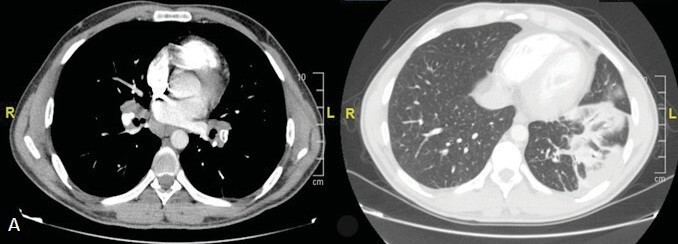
Pulmonary CT angiography (A)
